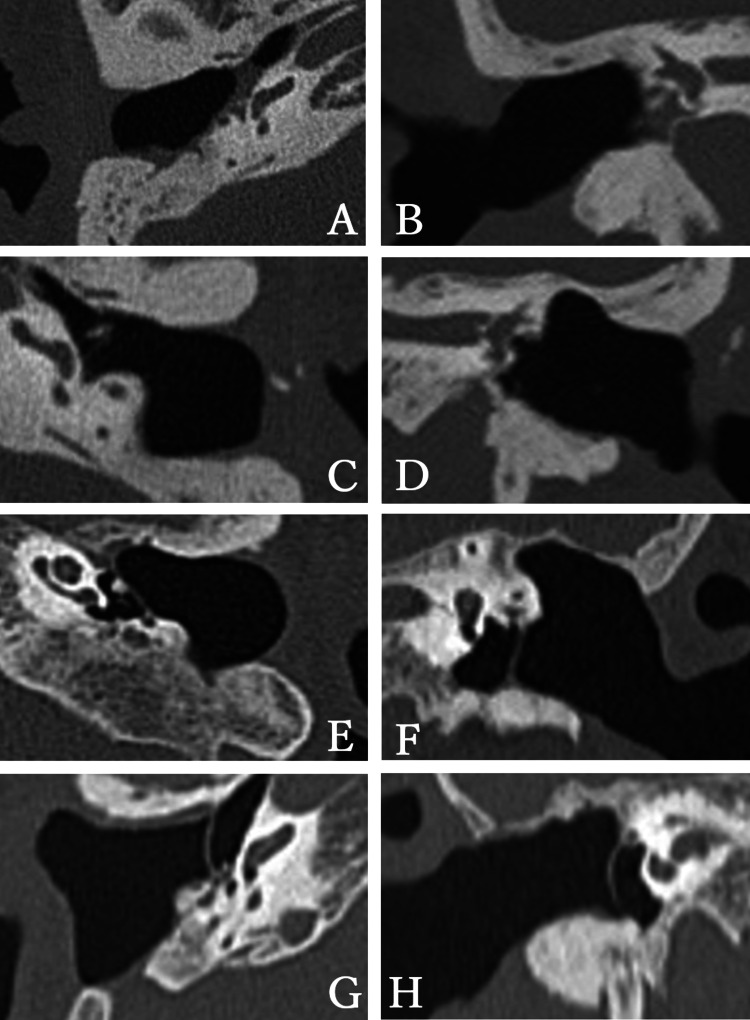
CT scan of cases 1, 2, and 3. (A-D) Preoperative temporal bone CT of case 1. The right ear (A: axial, B: coronal). The left ear (C: axial, D: coronal). (E, F) Preoperative temporal bone CT in case 2. Left ear (E: axial, F: coronal). (G, H) Preoperative temporal bone CT in case 3. Right ear (G: axial, H: coronal).
